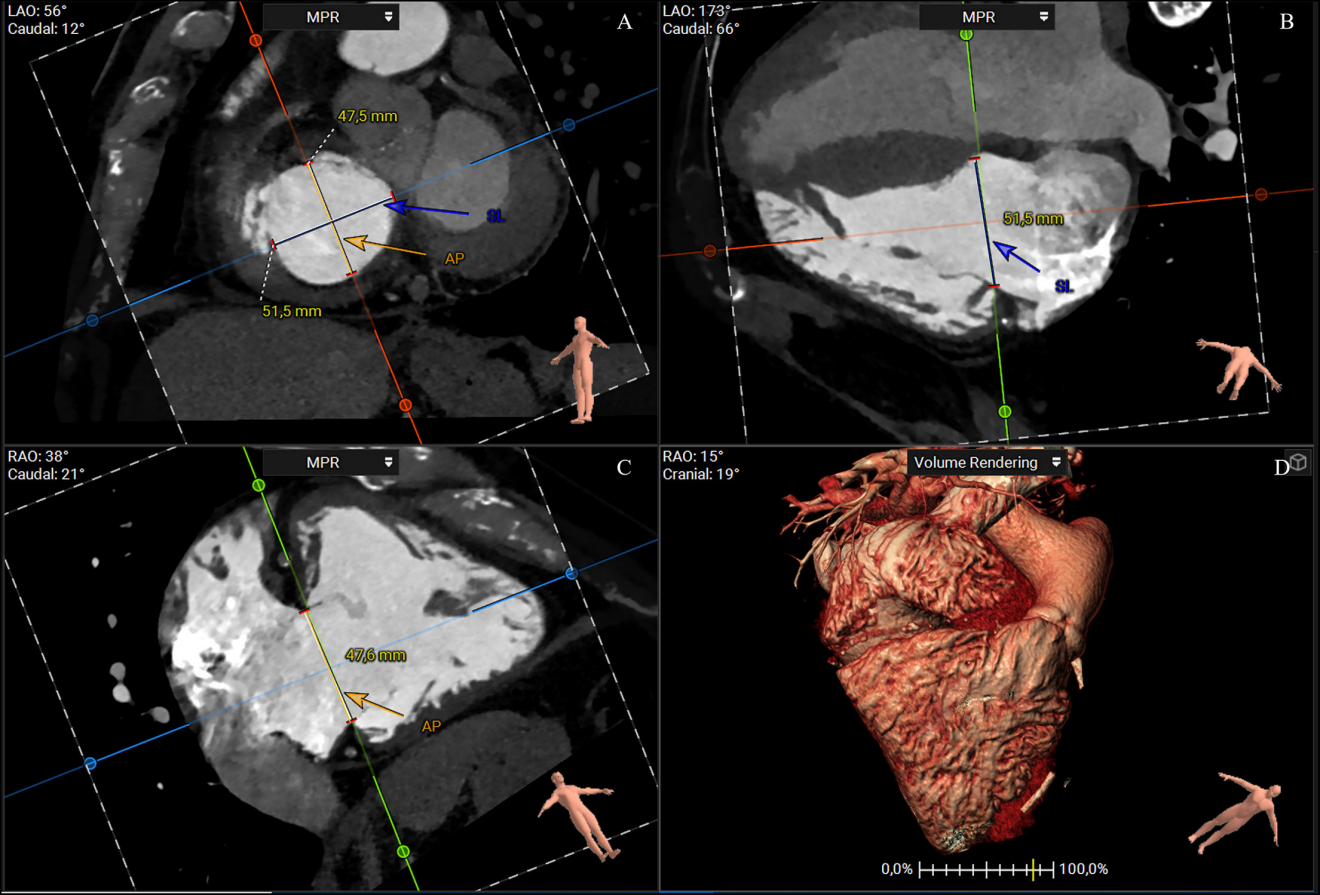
CT multiplanar reconstruction showing the short-axis plane at the annular level (A), the derived four chamber (B), two chamber (C), and volume rendering of the right chambers (D). The annulus septal-lateral diameter is measured in septal to lateral direction (A, blue line) and coincides with the annulus measurement in the four-chamber view (B). The anteroposterior diameter is orthogonal at the previous one (A, orange), and coincides with the measurement in the two-chamber view (C) (3mensio Structural Heart; Pie Medical Imaging, Maastricht, The Netherlands). AP, anteroposterior; SL, septal-lateral.
